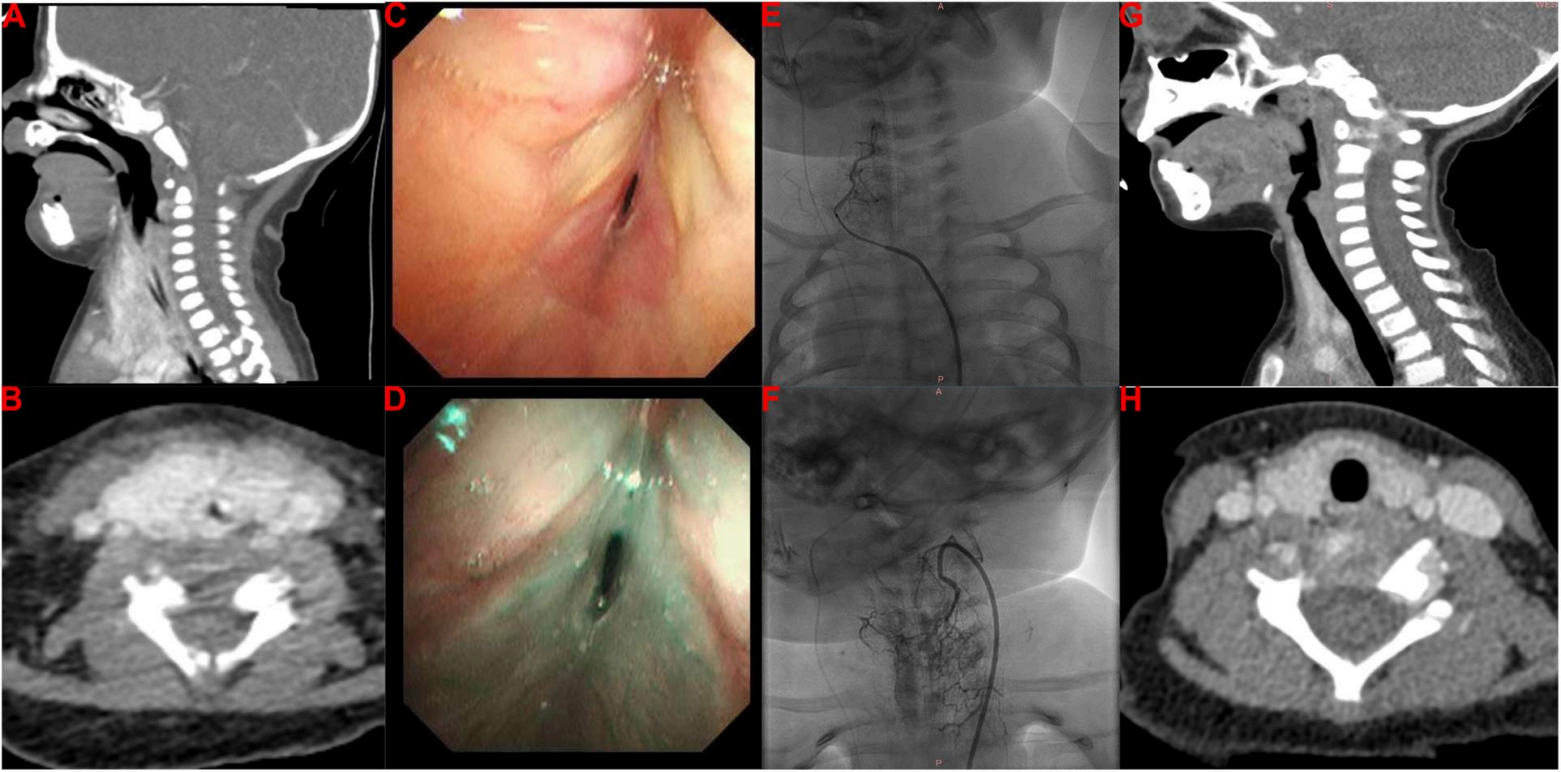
Imaging findings of our patient with infantile hemangioma with airway obstruction. (A,B) Contrast-enhanced CT imaging reveals a thickening of circumferential tissue with contrast enhancement around the subglottic area. (C,D) Flexible bronchoscopy identifies IHs in the airway and an airway obstruction. (E,F) Transcatheter interventional sclerotherapy is performed. (G,H) Repeated contrast-enhanced CT reveals that the circumferential tissue thickening with contrast enhancement around the subglottic area was resolved with the treatment.
